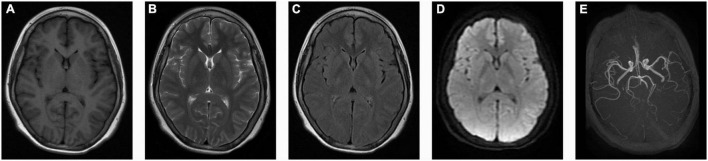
No abnormalities in the patient’s brain MRI and magnetic resonance angiography (MRA). (A) Axial T1-weighted images (T1WI); (B) Axial T2-weighted images (T2WI); (C) Axial T2 fluid attenuated inversion recovery sequence images (Flair); (D) Diffusion-weighted imaging (DWI); (E) Magnetic resonance angiography (MRA) images.
